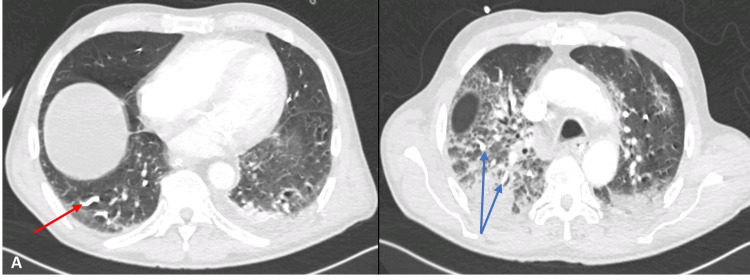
Chest CT scan showing embolization of NB2CYA A: 2 cm thrombus at subsegmental arteries (red arrow). B: Several thrombus and lung opacities (blue arrows). CT: computed tomography, NB2CYA: N-butyl-2-cyanoacrylate
